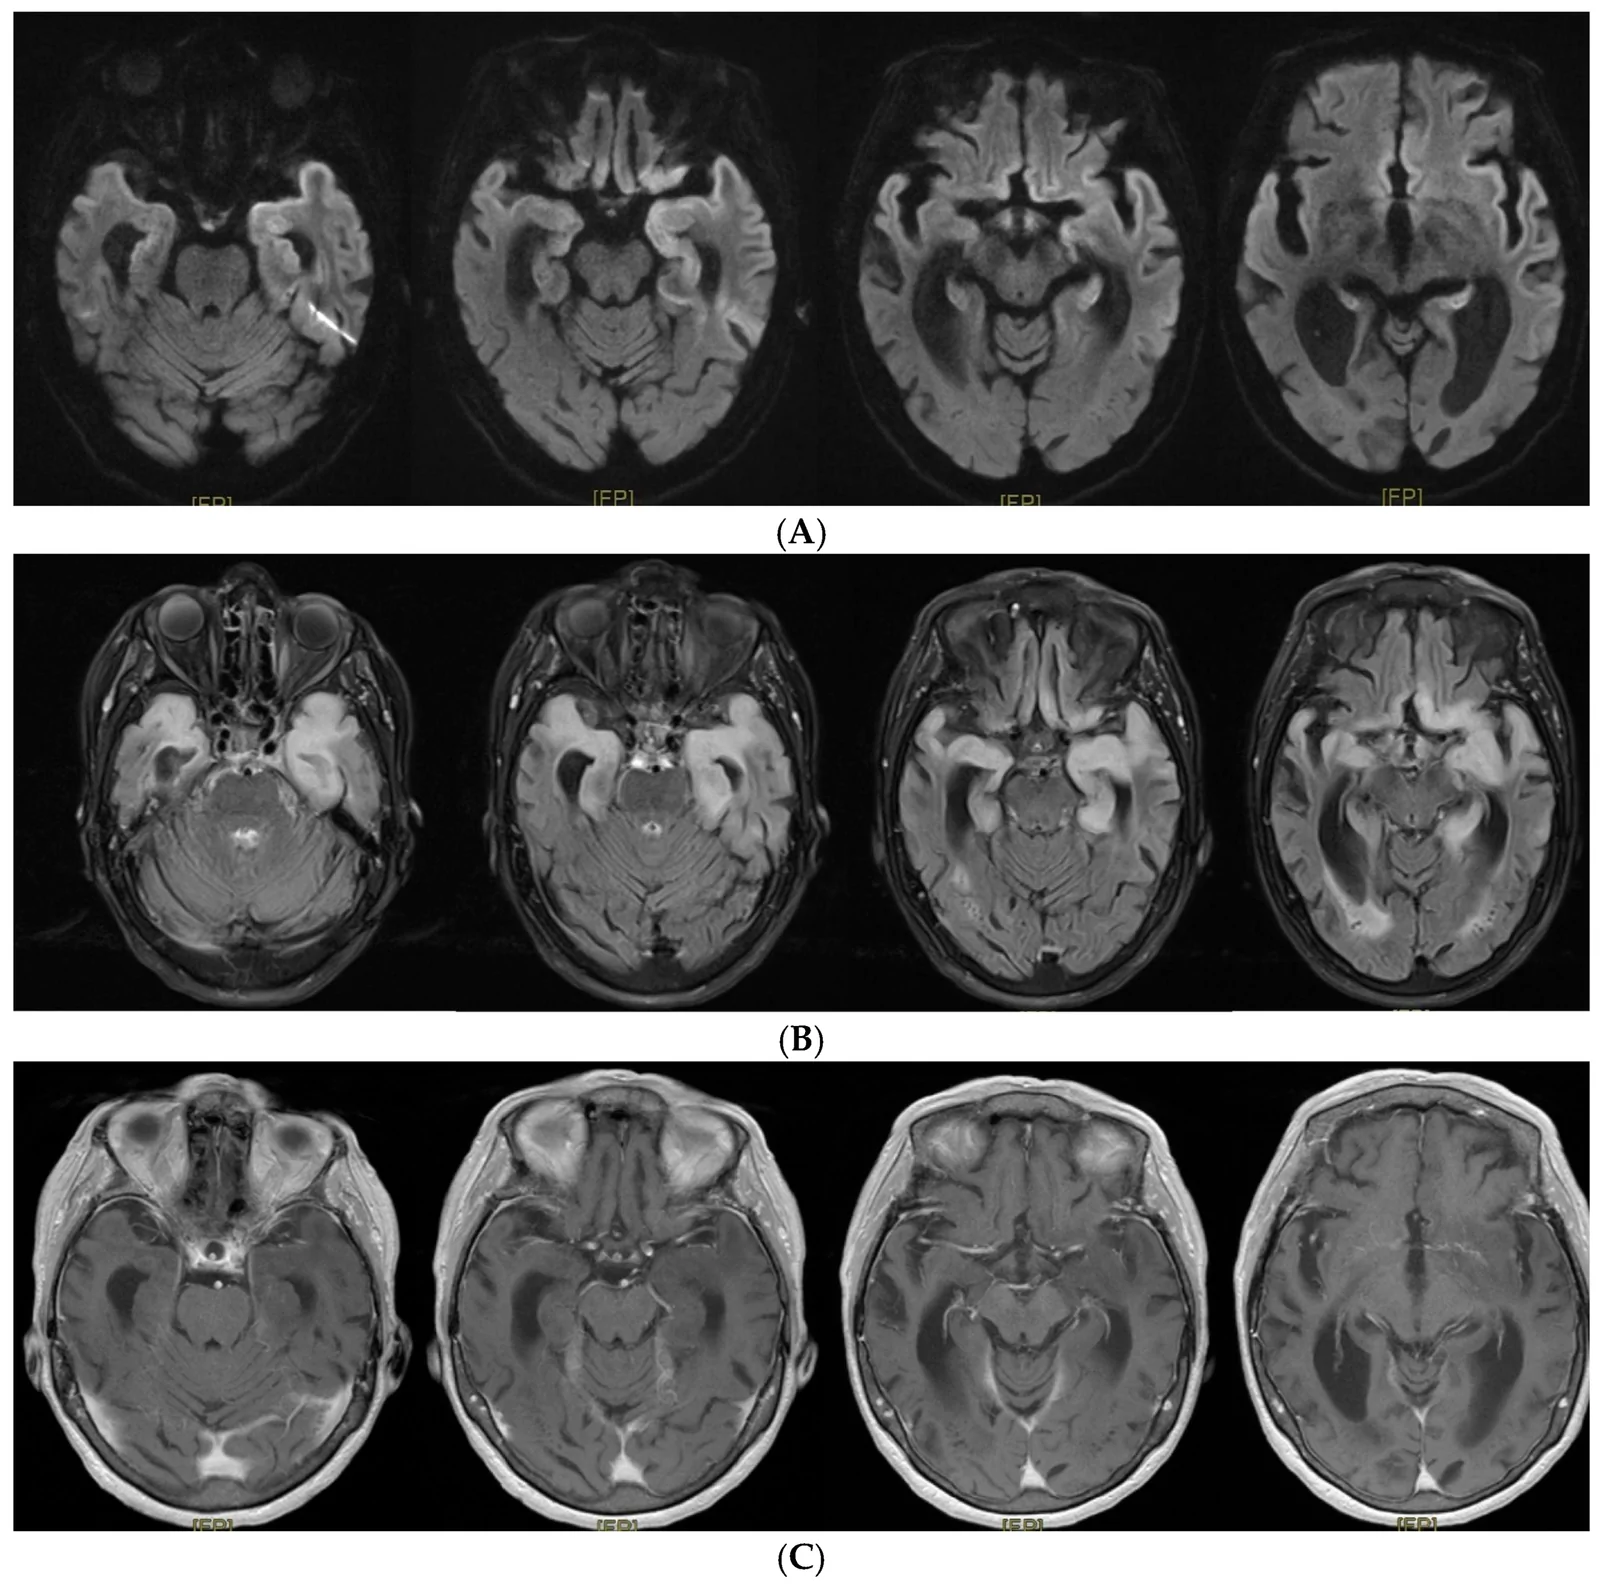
Magnetic resonance imaging (MRI) of the patient: (A) Diffusion-weighted imaging (DWI) MRI images. Bilateral cortical diffusion restriction involving the temporal lobes, insular cortices, and basal regions of the frontal lobes, with additional involvement of the mesial temporal structures, including the hippocampi and amygdala, as well as the anterior cingulate gyri, with a clear predominance on the left side. (B) Fluid-attenuated inversion recovery (FLAIR) MRI images. Hyperintense lesions involving both temporal lobes and the insular cortices, with involvement of the mesial temporal structures, hippocampi, amygdala, orbitofrontal cortex, and anterior cingulate gyri, more pronounced on the left side. (C) Post-contrast T1-weighted MRI images. No pathological contrast enhancement was observed following intravenous administration of a gadolinium-based contrast agent.
